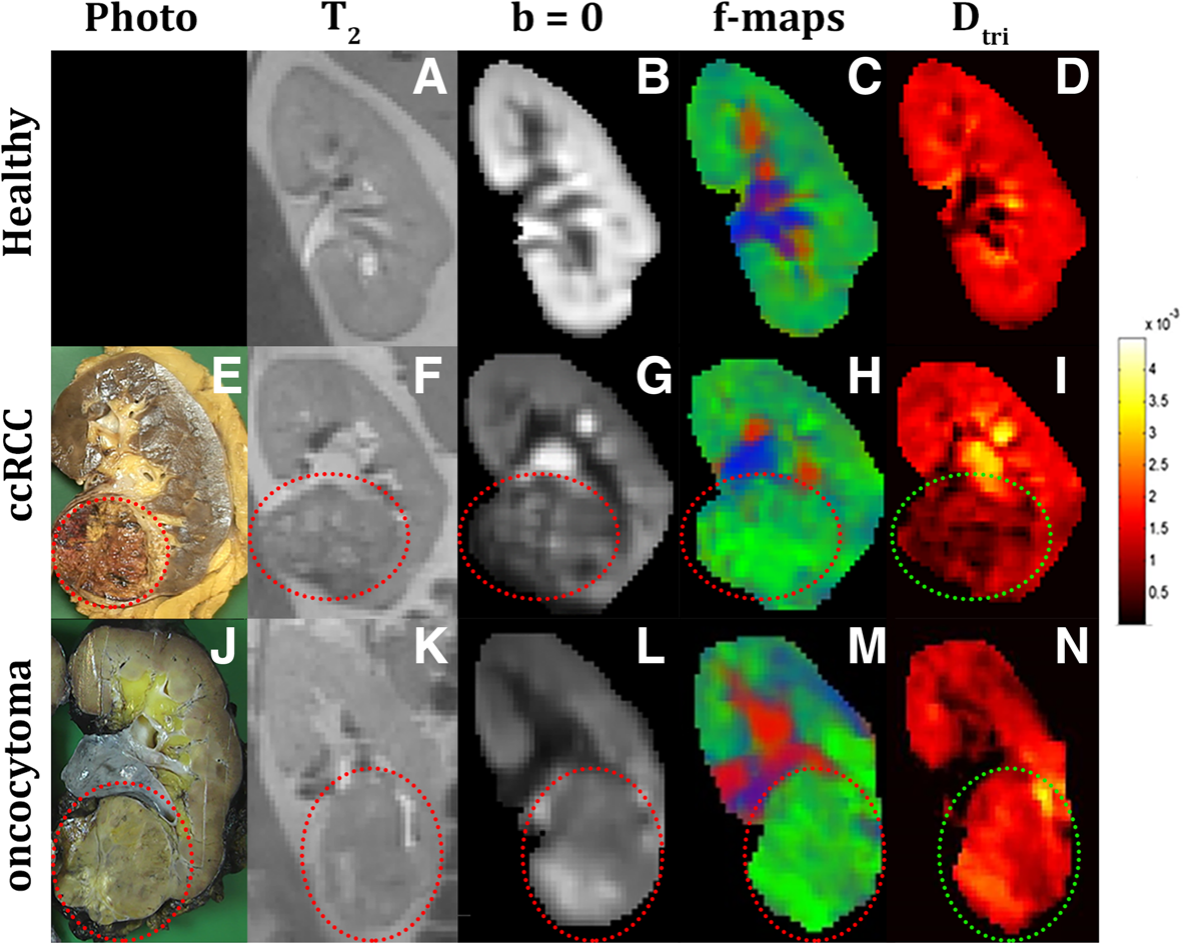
Diffusion-derived parameter maps of an unaffected kidney (a-d), a kidney with renal cell carcinoma (e-h), and a kidney with oncocytoma (i-l). First row (e, j): gross appearance of the whole kidney or tumour after nephrectomy, (a, f, k): anatomical reference (after processing) (T_2_-TSE), second row (b, g, l): the unweighted image of the diffusion scan after motion correction and image registration (DWI-b0), third row (c, h, m): a merge of the fraction maps from the tri-exponential fit red = *f*_*fast,*_ blue = *f*_*interm*,_ green = *f*_*slow*_ (1- *f*_*interm*_ - *f*_*fast*_), fourth row (d, i, n): diffusion coefficient from the tri-exponential fit, (*D*_*tri*_)
